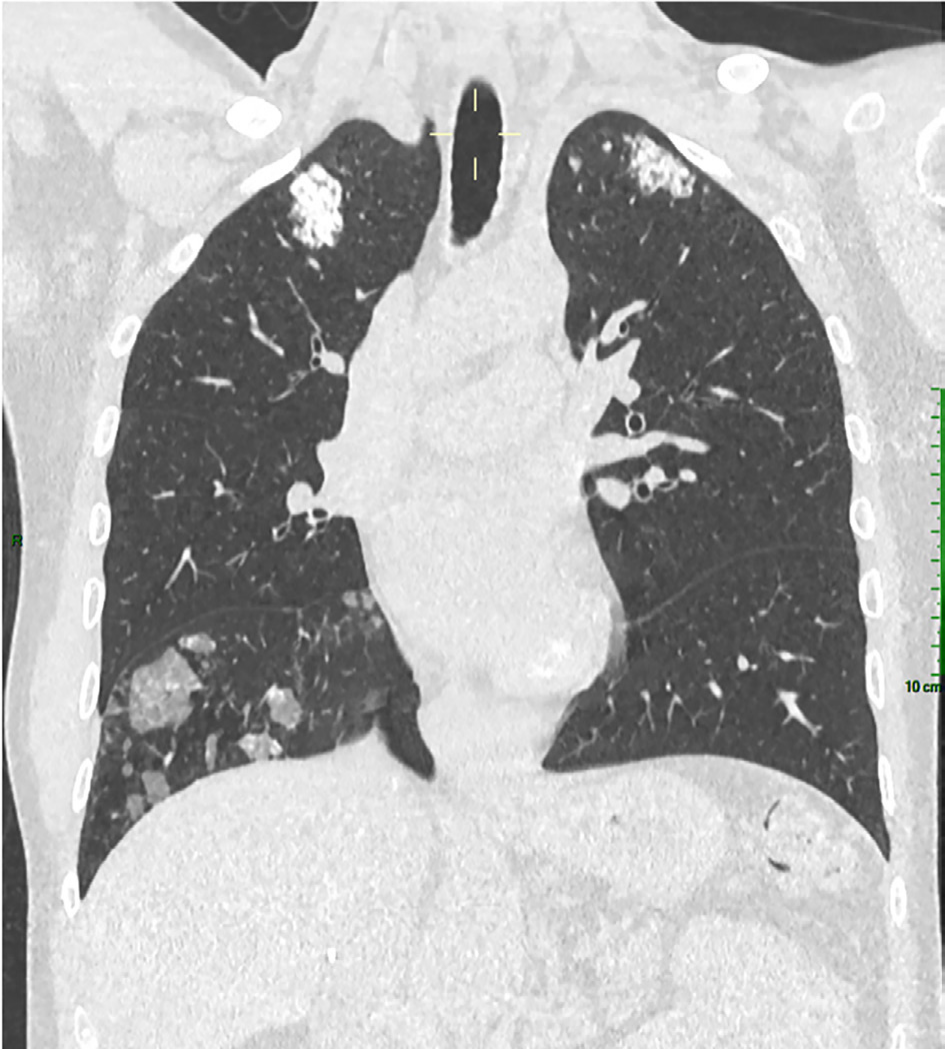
Coronal chest CT with bilateral ground‑glass nodular opacities, partially calcified, predominant in the superior regions.
